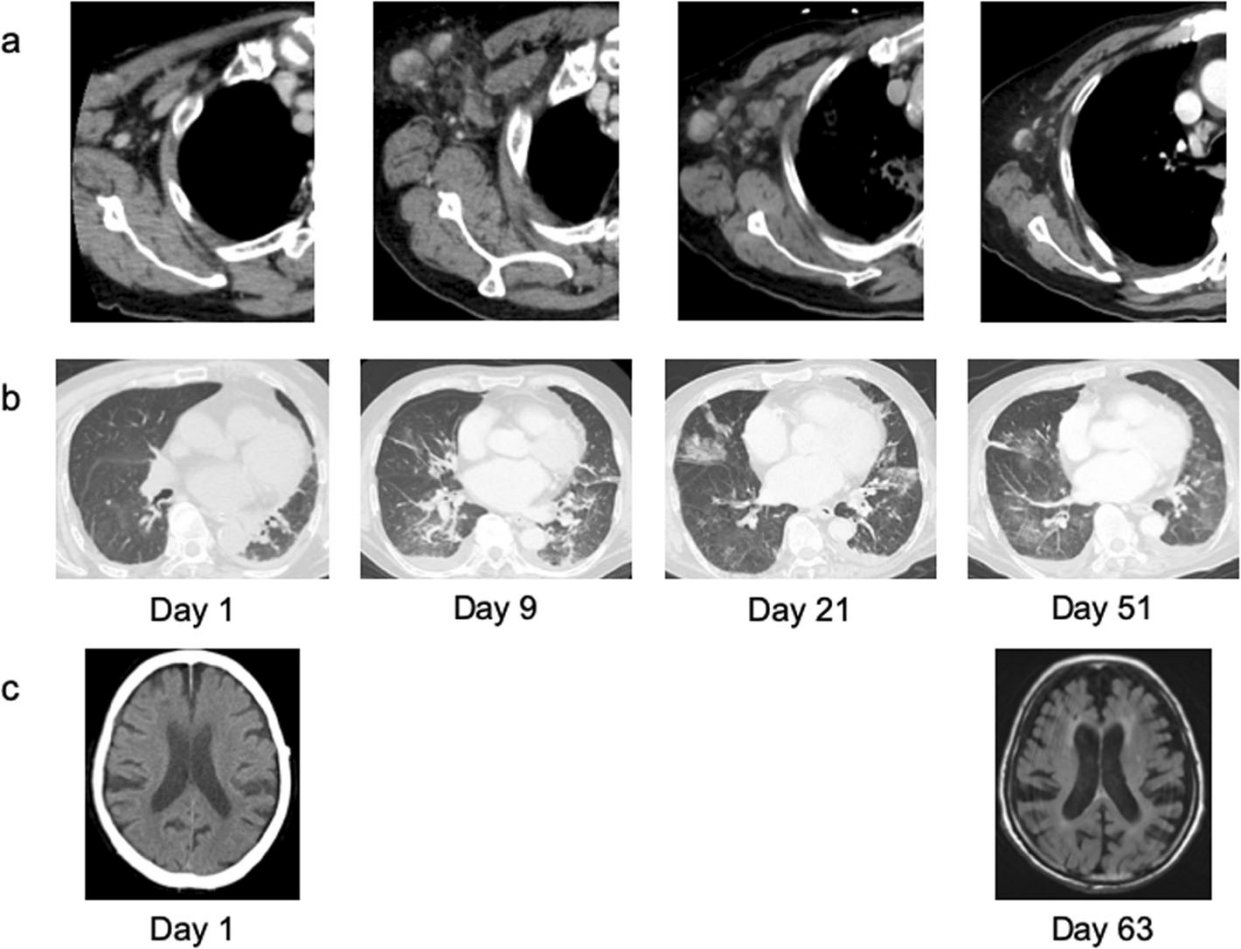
Chest and brain imaging. (a) Right axillary lymphadenopathy gradually reduced. (b) Chest CT showed diffuse ground glass opacity on days 9, 21, and 51. (c) Head CT (Day 1) and MRI (Day 63) showed no remarkable findings except cerebral atrophy
